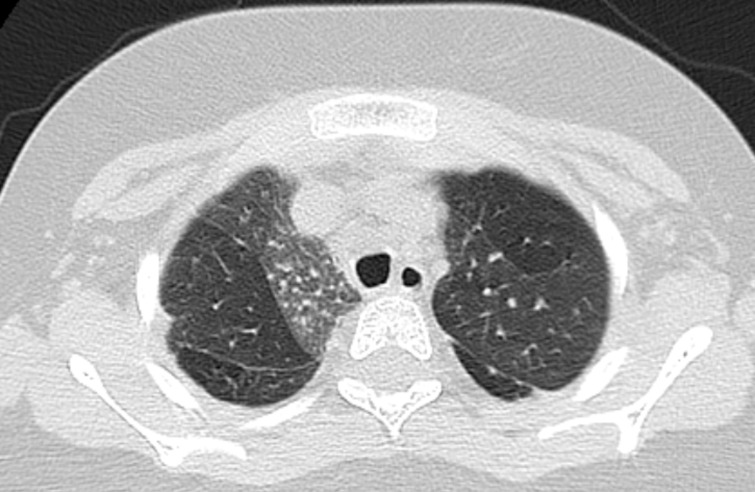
high resolution computed tomography of the chest (axial view)
